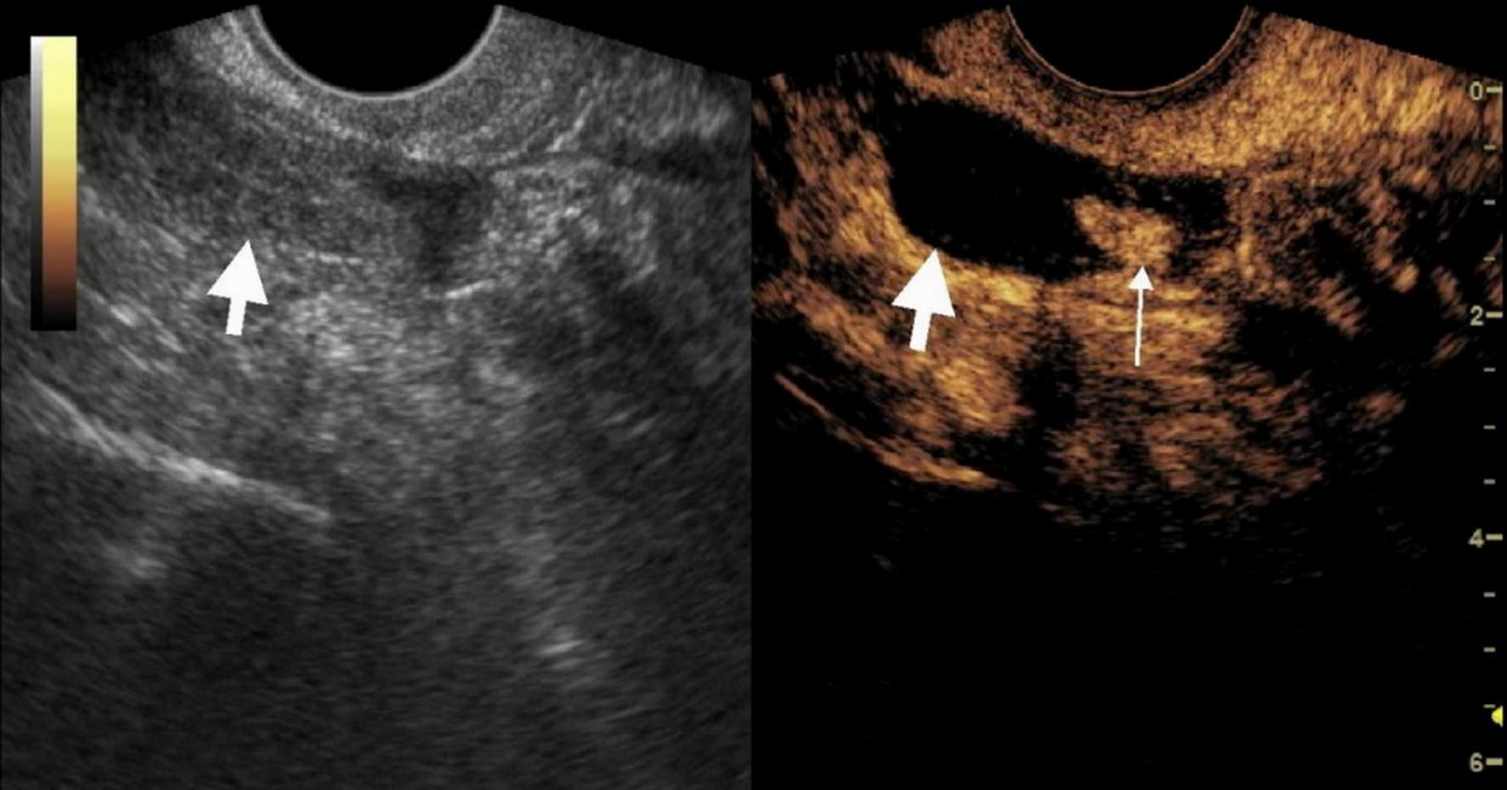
Transvaginal contrast enhanced ultrasound(CEUS) showed the longitudinal section of the fallopian tube. The patient was a 39-year-old woman who came to the hospital for amenorrhea and vaginal bleeding. Her blood HCG level was 806 mIU/mL. The time interval between CEUS and blood HCG measurement was less than 6h. Ultrasound examination found a low to medium echoic mass measuring 3.6cm × 1.8cm in the right adnexal region (left figure). The CEUS showed heterogeneous enhancement of the mass (right figure). The thick arrow indicated the dilated fallopian tube, and the thin arrow indicated the enhanced villous tissue. The non-enhanced area within the mass was confirmed intraoperatively to be blood clots within the fallopian tube. The villous tissue size visible during the operation was 0.5cm × 0.5cm, similar to the size measured by ultrasound (0.8cm × 0.5cm). The enhancement pattern of this patient was non-annular enhancement.This patient was confirmed by surgery to have a pregnancy in the ampulla of the right fallopian tube. Transvaginal contrast enhanced ultrasound (CEUS) showed the longitudinal section of the fallopian tube
